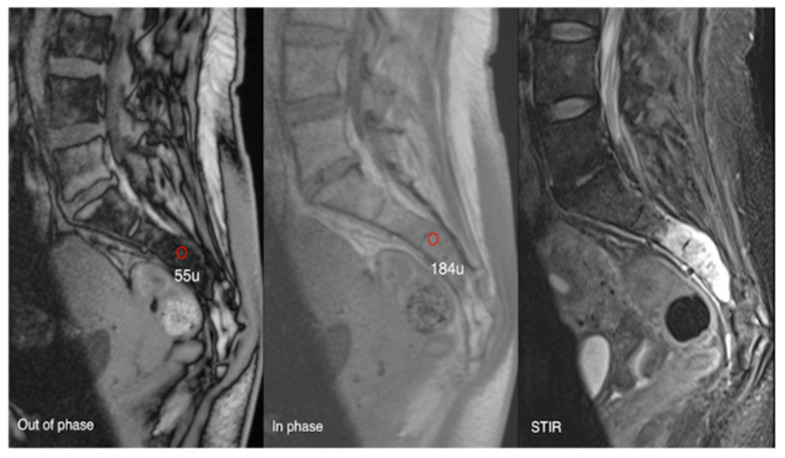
Aggressive sacral haemangioma with soft tissue mass. Out-of-phase, in-phase, and STIR sagittal MRI spine as labelled on images (bottom left), showcasing the expansile nature of the lesion but also the internal fat content as demonstrated by >20% signal dropout of signal intensity values (55 SI vs. 184 SI) on the out-of-phase vs. in-phase sequence.
